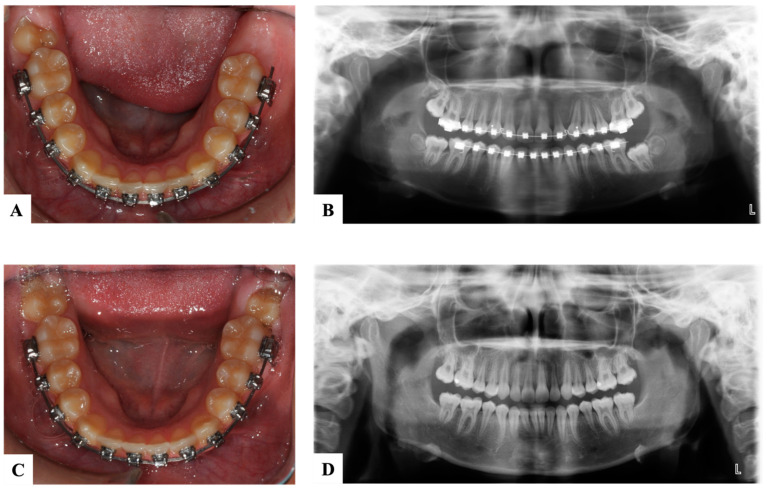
Ectopic mandibular left second permanent molar (#37) with impacted third molar (#38). (A,B). Occlusal photograph and panoramic radiograph before the treatment of the second molar (#37). (C). Occlusal photograph 11 days post-surgical uprighting of the second molar (#37) and extraction of third molar (#38). (D). Panoramic radiograph after the treatment. (Courtesy of Dr. S. Alfuriji.)
